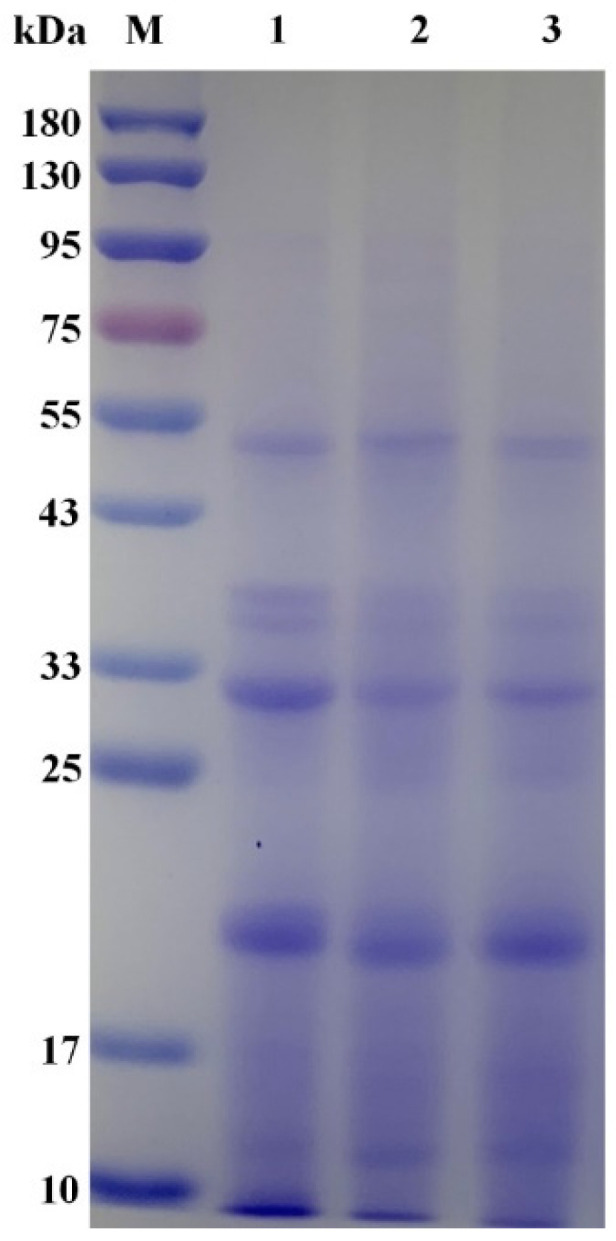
Effects of ultrasonic treatment and germination on the protein profiles of quinoa. (M, standard protein marker; lane 1, 0 W–0 h; lane 2, 0 W–24 h; lane 3, 100 W–24 h).
